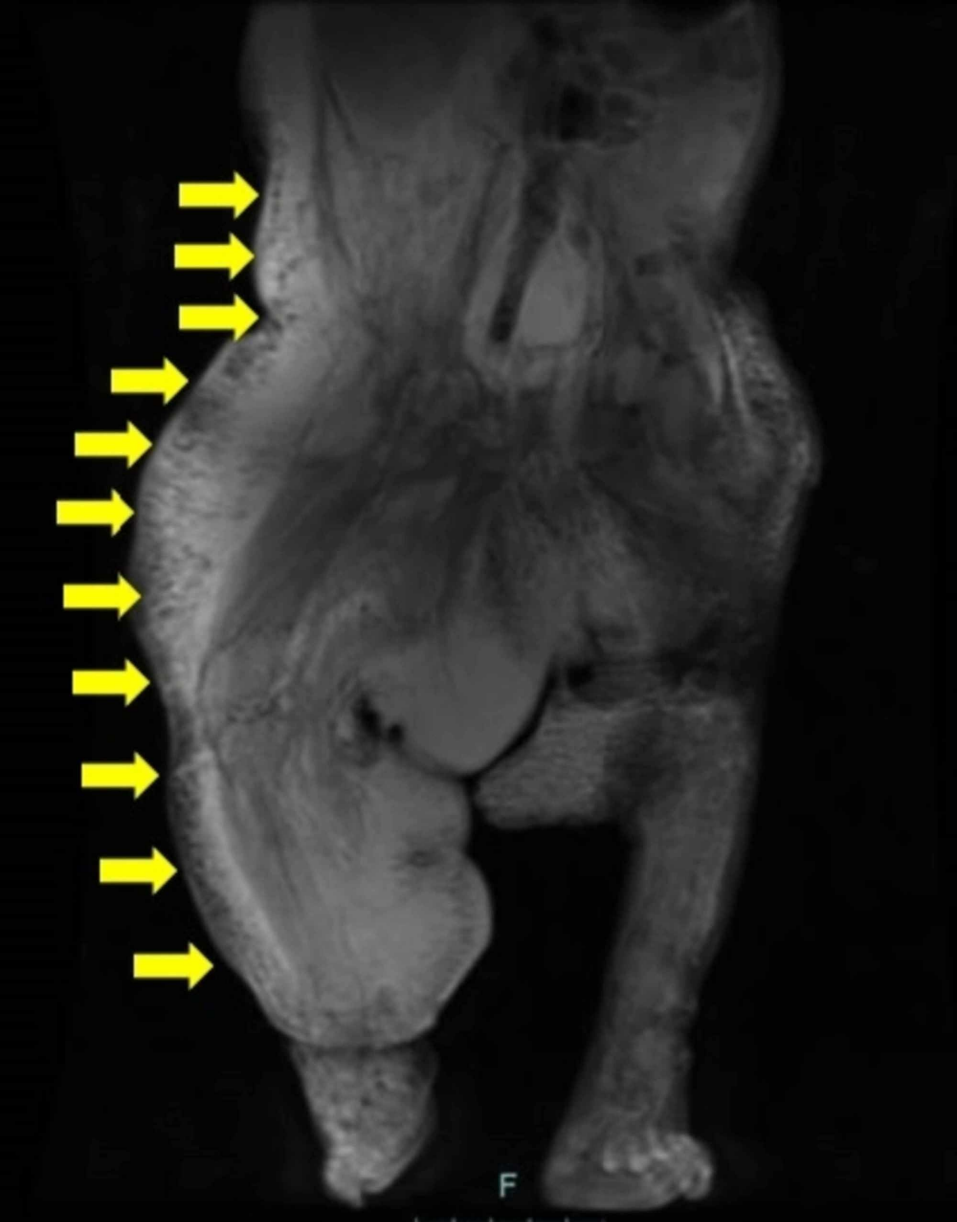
Pre-gadolinium T2-weighted coronal magnetic resonance imaging (MRI) scan showed white lymphatic malformations in most of the right side of the body (yellow arrows)
